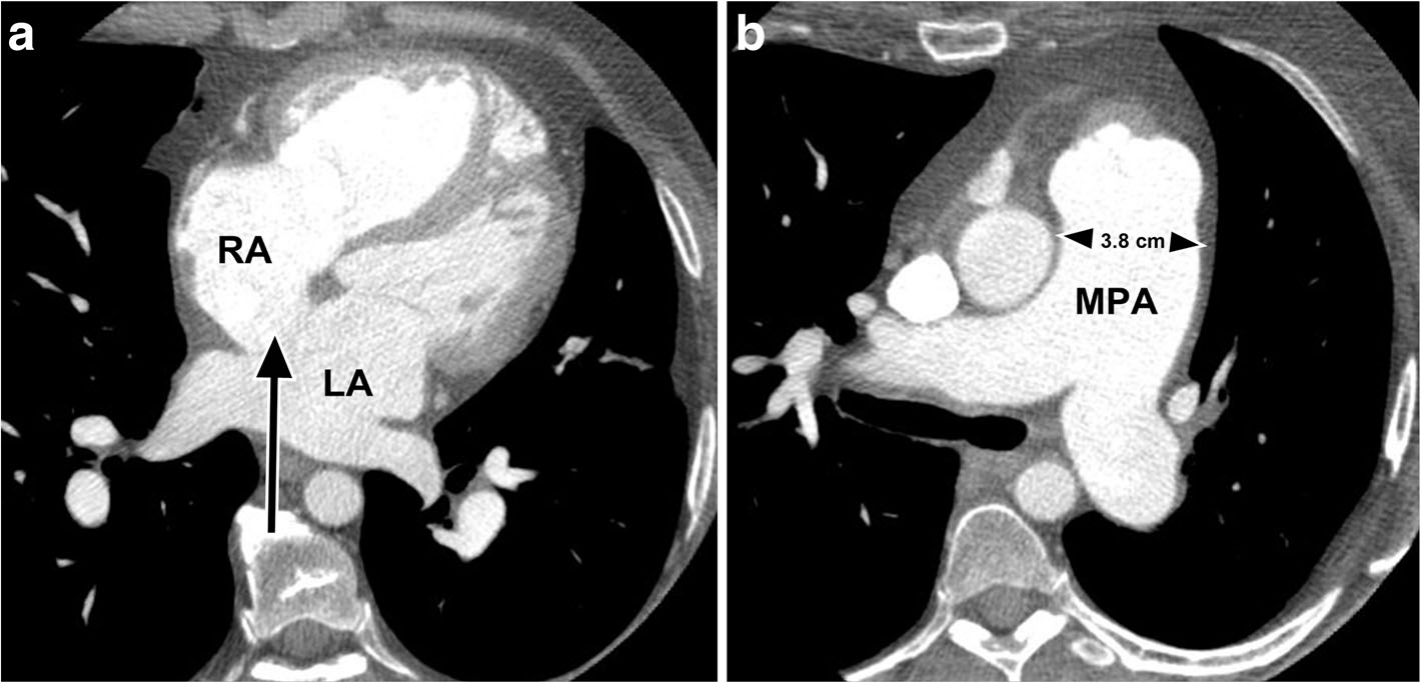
a Large ostium secundum ASD (black arrow) in a 51-year old female who was being investigated for cause of pulmonary hypertension. *RA* right atrium, *LA* left atrium. b The patient also had large central pulmonary arteries demonstrated by thoracic CT. *MPA* main pulmonary artery
